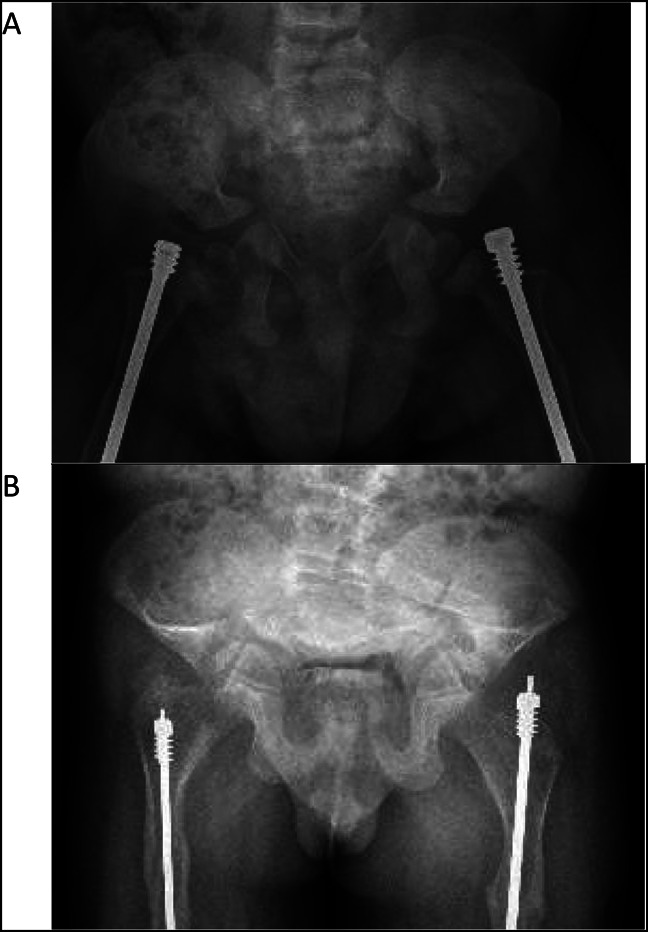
Radiograph of A, AP pelvis at 2 years and (B) AP pelvis at 10 years demonstrating marked progression of the patient's pelvic deformation and acetabular protrusio.
